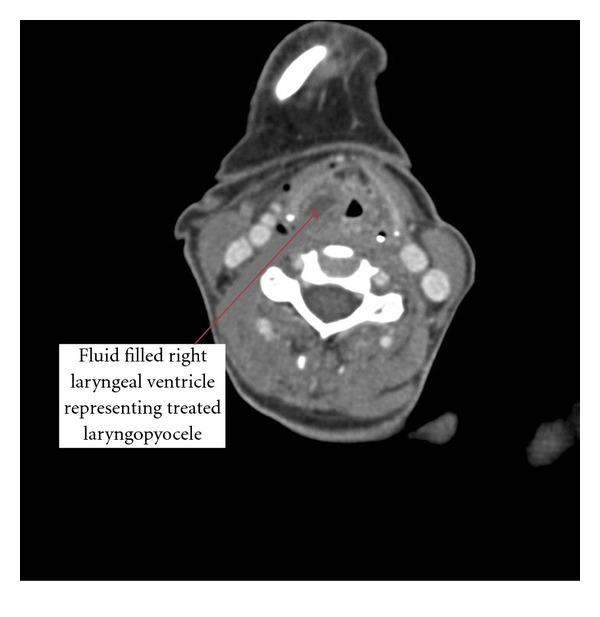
CT image immediately postoperatively with evidence of the marsupialised laryngopyocele on the right.
